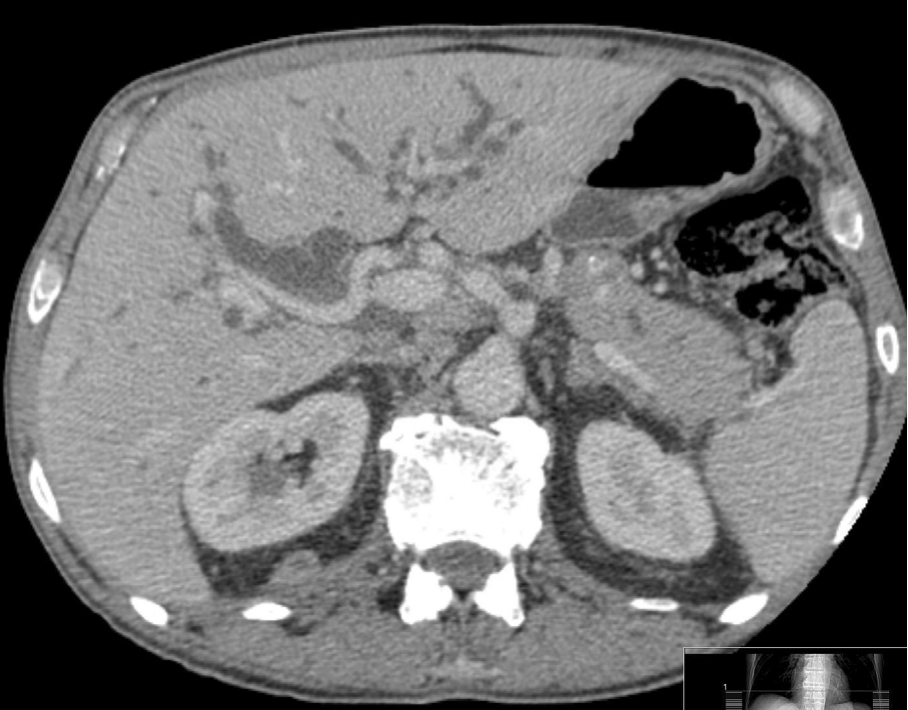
Axial computed tomography image showing intrahepatic duct dilatation.
